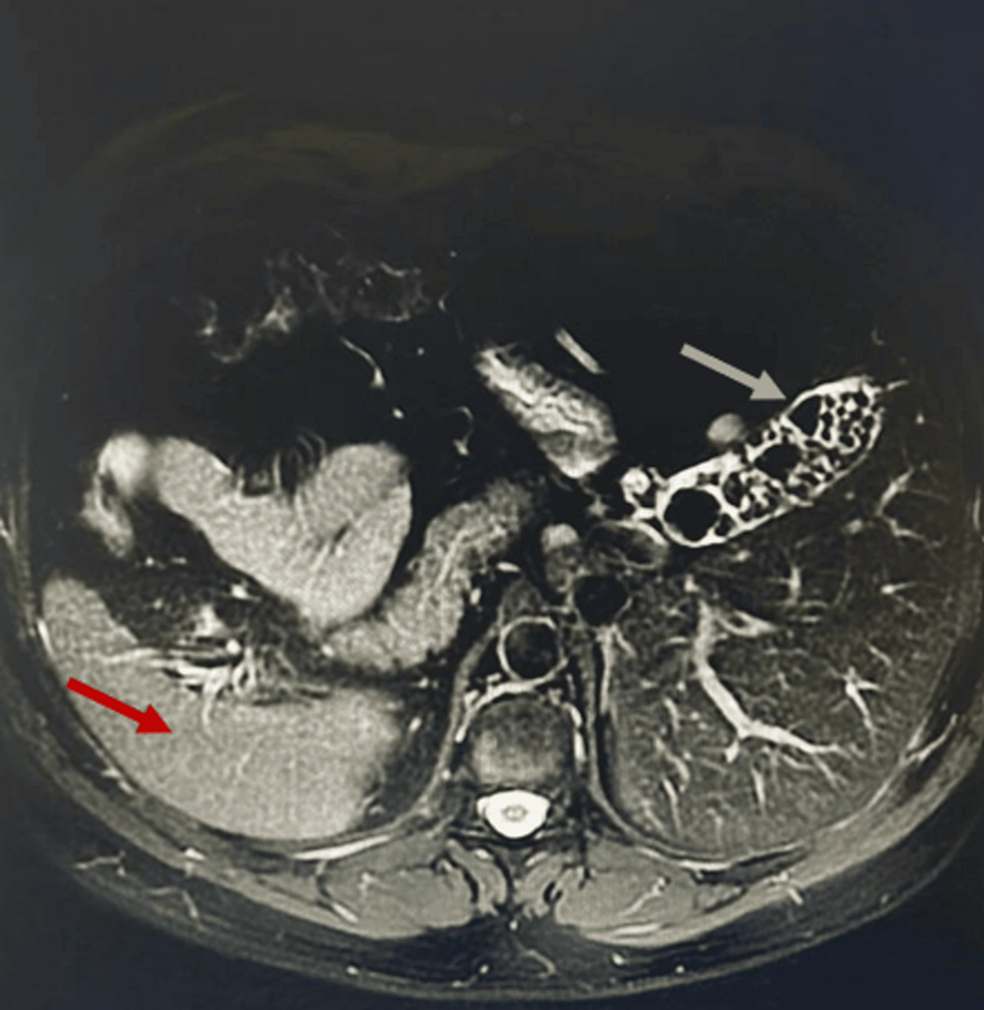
MRI, T2 weighted, axial plane Grey arrow: Calculous gallbladder Red arrow: Right-sided spleen
